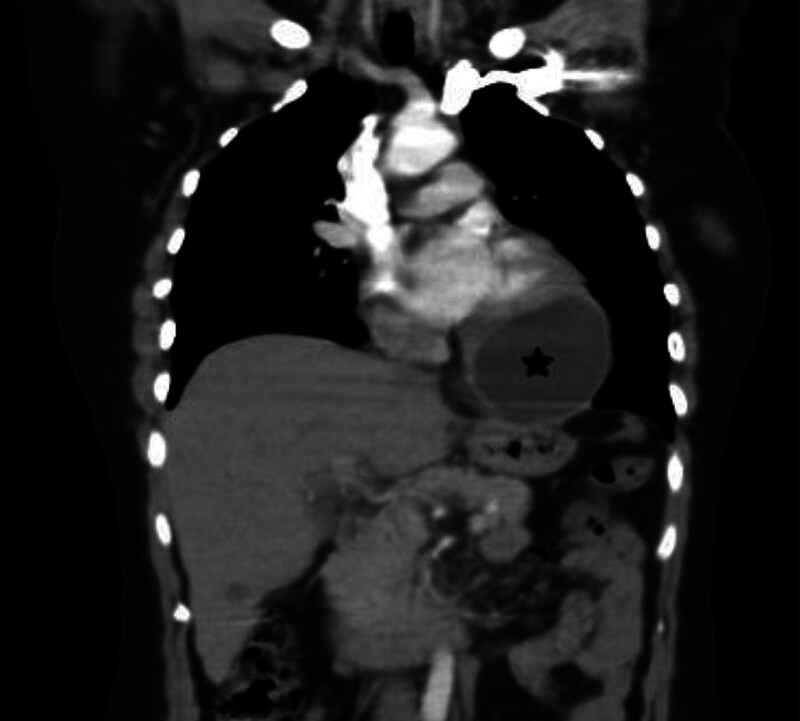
Coronal contrast-enhanced CT scan shows the cardiac hydatid cyst at the left ventricular apex (black star). CT = computed tomography.
